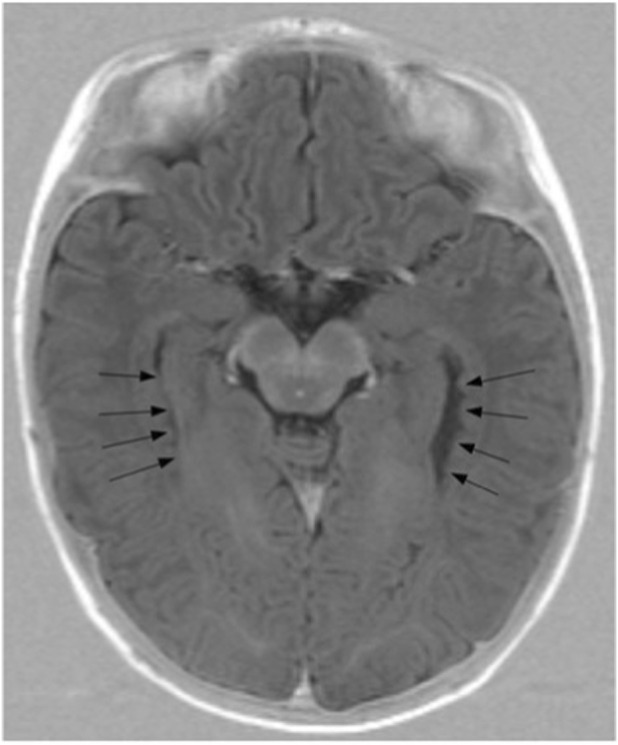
Axial MRI of T1 at the level of the temporal horns demonstrating multiple nodules of heterotopic grey matter lining the lateral walls of the temporal horns of the lateral ventricles bilaterally (black arrows).
